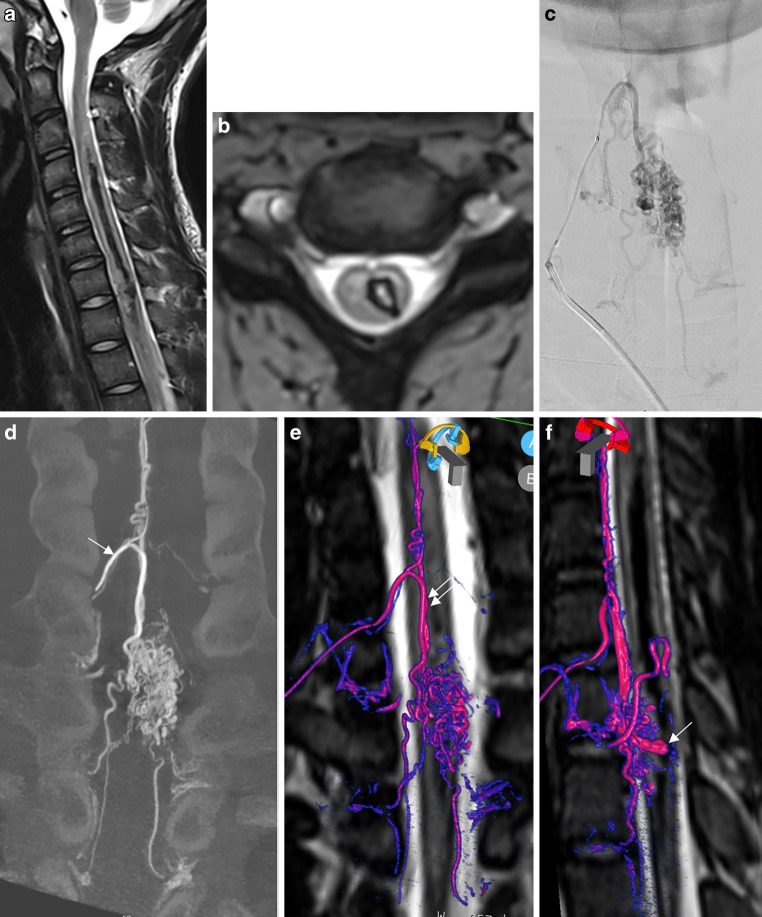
Ruptured spinal arteriovenous malformation (AVM) with a large intranidal aneurysm. a T2W sagittal image demonstrating extensive left-sided hematomyelia. b Hemoflash demonstrating the extension of the hematoma within the left side of the spinal cord. c (DSA anterio posterior projection). d (coronal reformated 3DRA) Superselective injection of the main radiculomedullary artery of the cervical enlargement (artery of Lazorthes, *arrow*) demonstrating a large nidus and multiple draining radicular veins. e, f Fusion of the three-dimensional rotational angiography (3DRA) and T2W spine magnetic resonance imaging (MRI) demonstrating the feeding radiculomedullary artery which supplies the anterior spinal artery (*double arrow*) which than feeds the AVM (*red/pink* for the artery and *purple* or *blue* for the draing vein). The intranidal aneurysm is localized within the central part of the myelon and the tip of the aneurysm is pointing towards the hematoma. Supplementary video demonstrates the fusion with an embedded 3D model of the AVM
